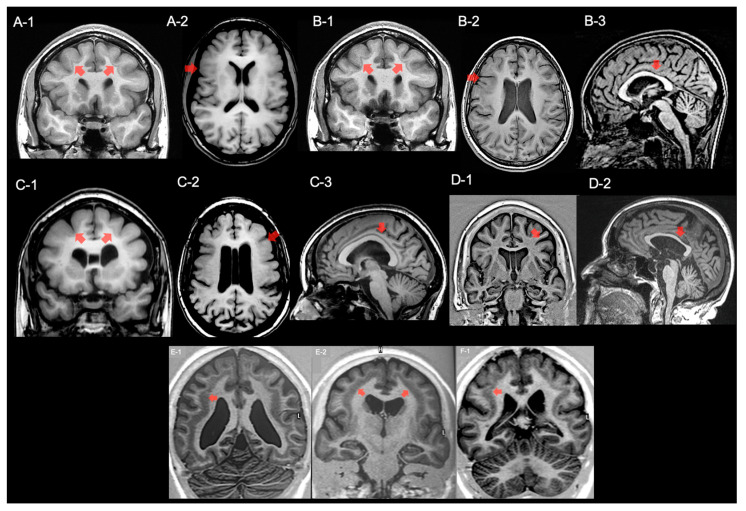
Brain MRI changes of Family 1, 2 and 3. Coronal (A-1) and axial (A-2) T1-weighted images of the proband (F1-III-1) of Family 1 showed a fronto-central SBH with mixed pachygyria (red arrows). Coronal (B-1), axial (B-2) and sagittal (B-3) T1 images of the F1-III-2 also showed a fronto-central SBH with mixed pachygyria together with a hypoplastic aspect of corpus callosum and cavum vergae (red arrows). Brain MRI (C-1–C-3) of the (F1-II-1) showed the same findings of the proband. Notice the dysmorphic aspect of corpus callosum (C-3, red arrow). MRI findings of the F1-I-1 with fronto-central SBH with mixed pachygyria and a dysmorphic aspect of corpus callosum as well (D-1,D-2). Coronal T1-weighted images of the proband (F2-II-1) of Family 2 show a bi-hemispheric fronto-parietal pachygyria with a thick SBH (E-1,E-2). Also notice the thick band of SBH of the proband (F3-II-1) of Family 3 (F-1, red arrow).
